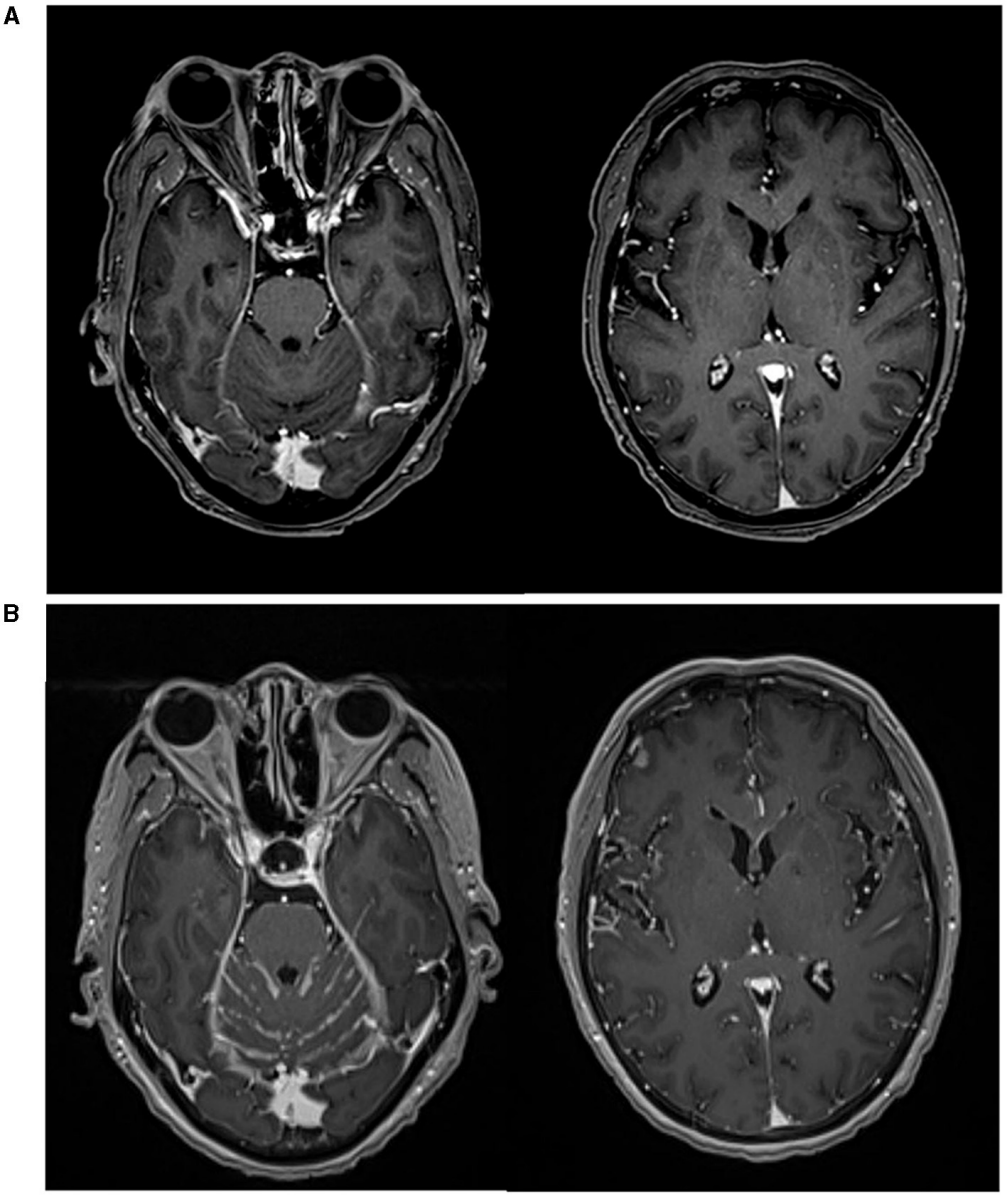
Brain MRI scans. (A) MRI image at the time of SCLC transformation. (B) MRI confirmed metastasis to the central nervous system (CNS).
